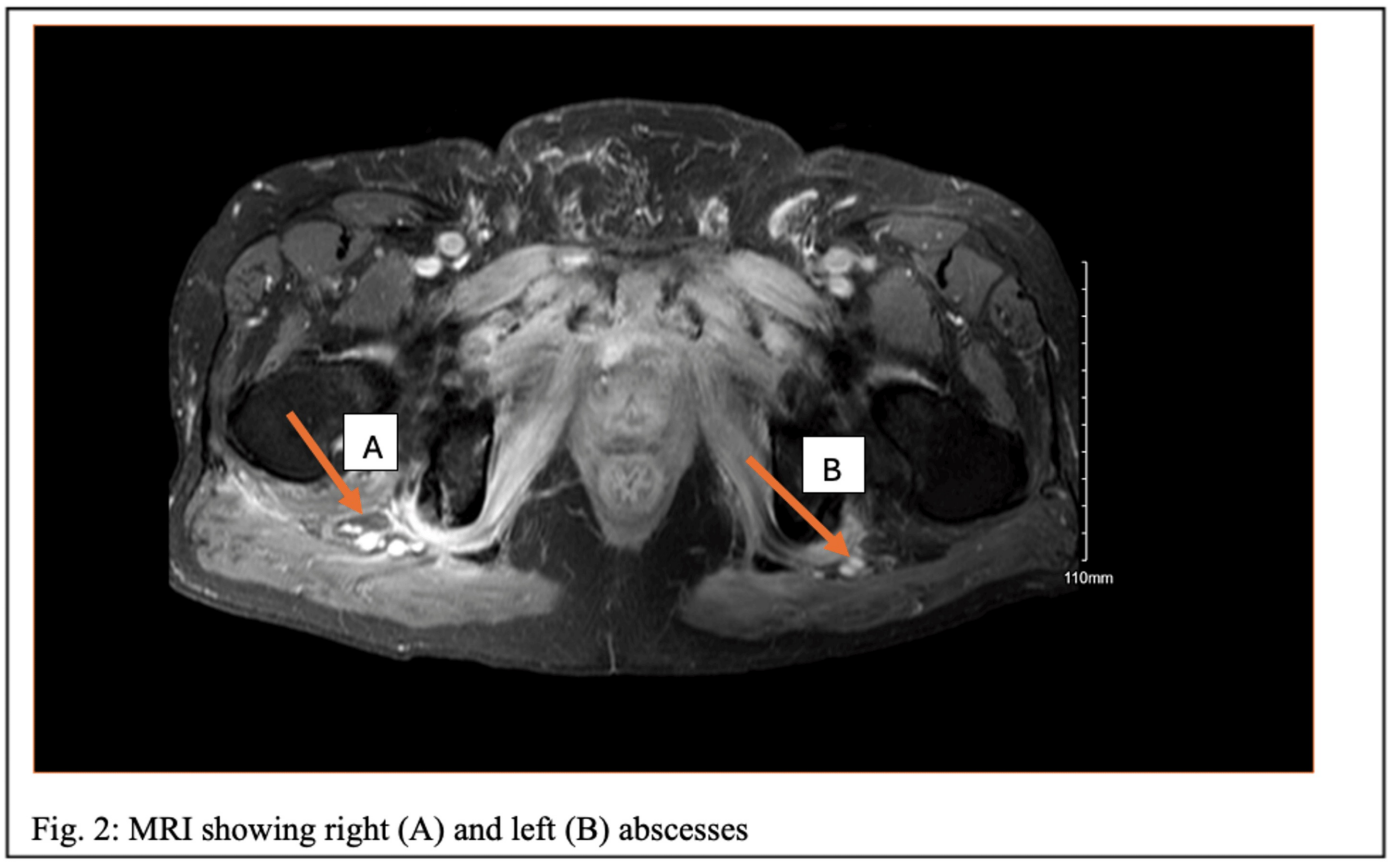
Axial T1-weighted MRI postcontrast showing right and left pelvic abscesses
